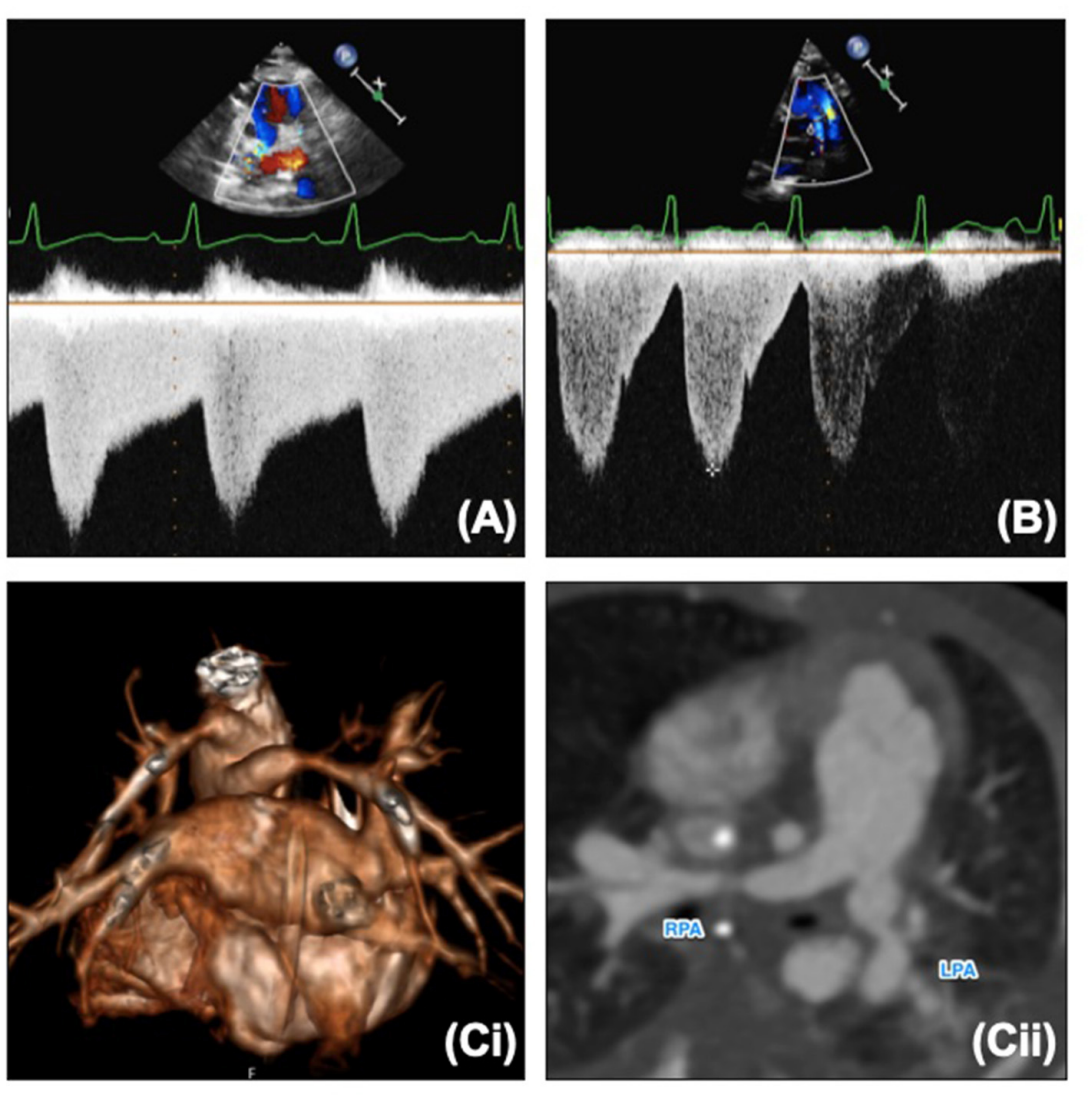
(A) Normal “sawtooth” pulmonary band Doppler pattern. (B) Abnormal “pulsatile” pulmonary band Doppler pattern. (C,i) 3D reconstruction of banded branch pulmonary arteries. (C,ii) Reformat of CT and banded branch pulmonary arteries. LPA, Left pulmonary artery; RPA, right pulmonary artery.
